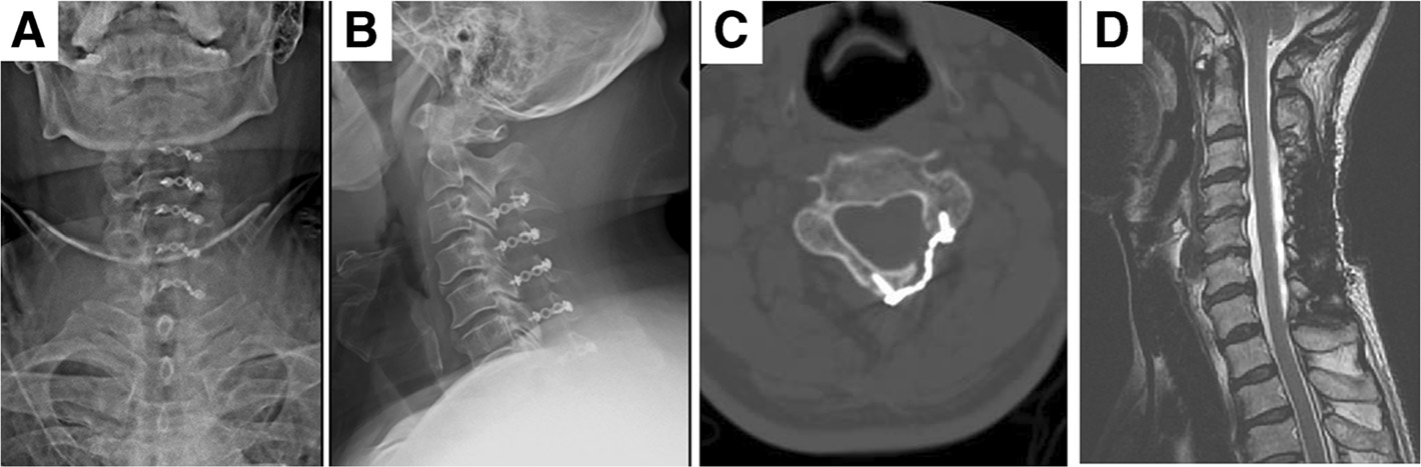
Postoperative radiographic follow-up. (A) AP view and (B) lateral view at postoperative 3 months. (C) CT scan at postoperative 6 months revealed well-positioned miniplate and screws and bone healing of hinge side. (D) MRI study at postoperative 12 months showed patent spinal cord without compression.
